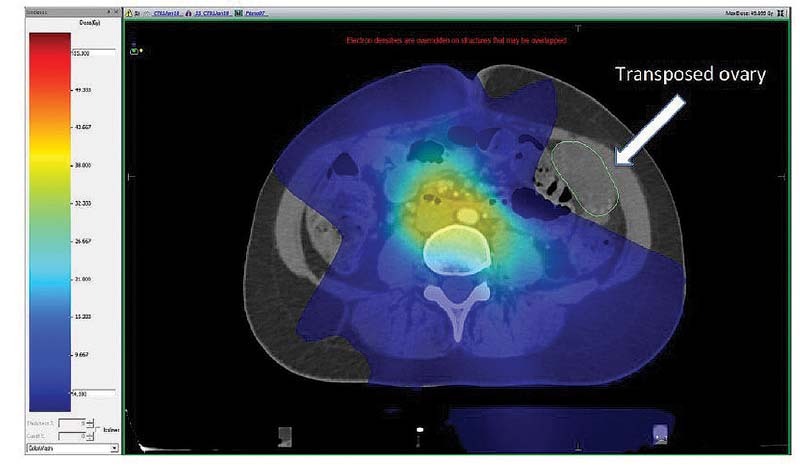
Computed tomography planning with volumetric arc therapy (VMAT) (Monaco TPS – version 5.51) of a 27-year-old patient in adjuvant radiation for cervical cancer. Isodose (blue color wash) of 4 Gy does not touch the transposed ovary (white arrow).
